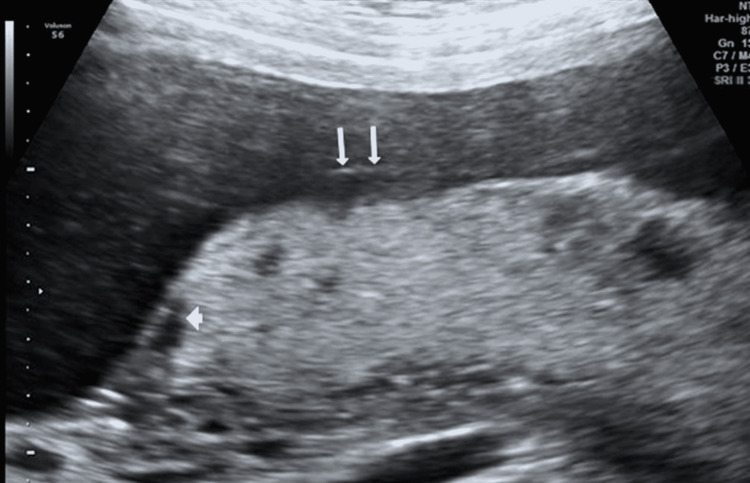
Ultrasonographic placental image at 17th week of gestation: subchorionic fibrin deposits (arrows) and blood pools (arrowhead) are observed.
